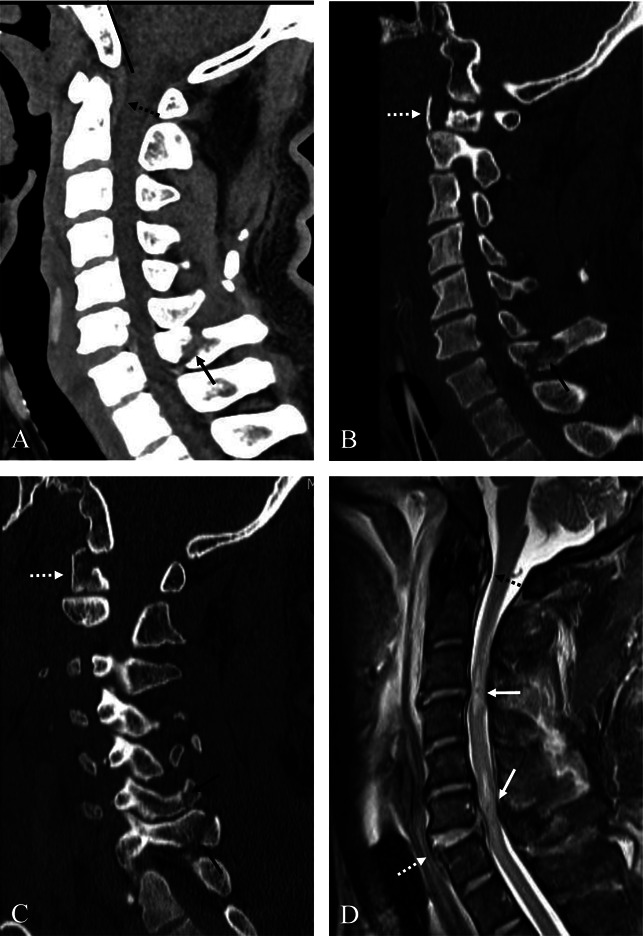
Sagittal reconstructed CT scans (A, B, and C) showing the direction of the Wackenheim line (dark line) behind the dens, indicating traumatic posterior atlanto-occipital dislocation (AOD), injury to the posterior ligament complex at the craniovertebral junction (CVJ) (dotted dark arrow), fracture of the C1 atlas (dotted white arrows), and fracture of the spinous process at C6 and C7 (dark arrows). Sagittal MRI (D) indicates traumatic intramedullary hemorrhage at C3-4 and C6-7 (white arrows), rupture of the anterior longitudinal ligament, disk, and posterior longitudinal ligament (dotted white arrows) at C3-4 and C6-7, the direction of the Wackenheim line (dark line) behind the dens, indicating traumatic posterior AOD and injury to the posterior ligament complex at the CVJ (dotted dark arrow).
